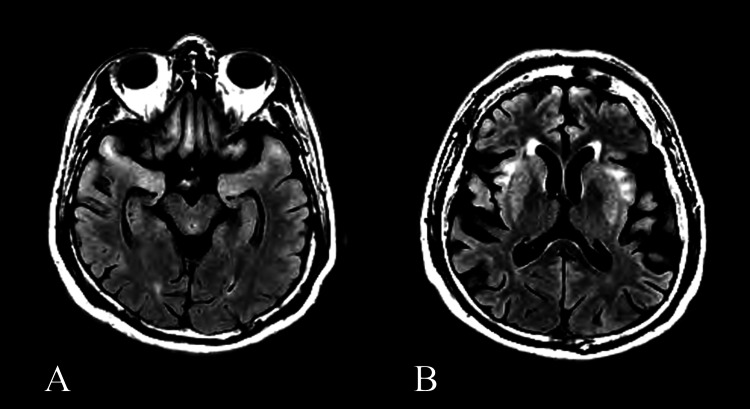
MRI findings in December 2019 A, B: axial T2W-FLAIR MRI taken in December 2019 depicting bilaterally symmetric hyperintensities in the (A) orbital frontal lobes, medial temporal lobes, (B) subinsular cortical areas, and basal ganglia. (B) A notable increase in cortical atrophy is observed in the frontal and temporal lobes T2W: T2-weighted: FLAIR: fluid-attenuated inversion recovery: MRI: magnetic resonance imaging
